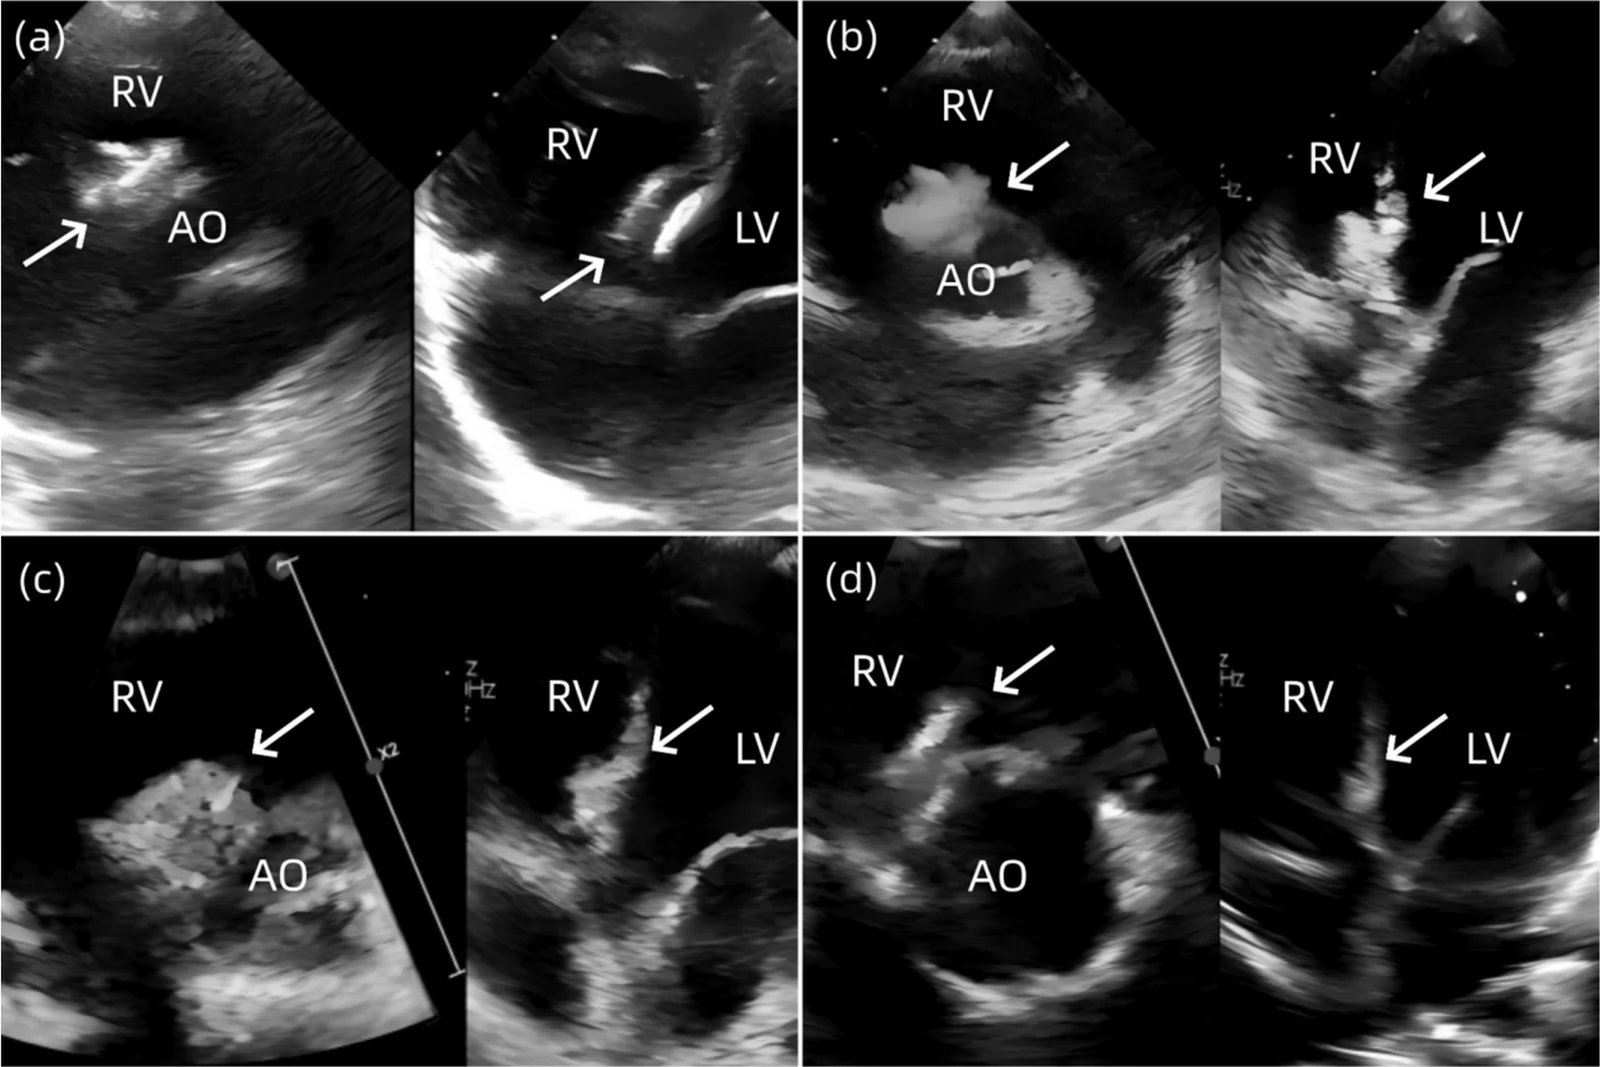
Serial echocardiographic changes of the bioabsorbable occluder at 1-year follow-up. (a–d): TTE short-axis view and five-chamber view were examined at 1-, 3-, 6-, and 12-month follow-up, respectively. Arrows indicate the occluder. AO, ascending aorta; RV, right ventricle; LV, left ventricle.
